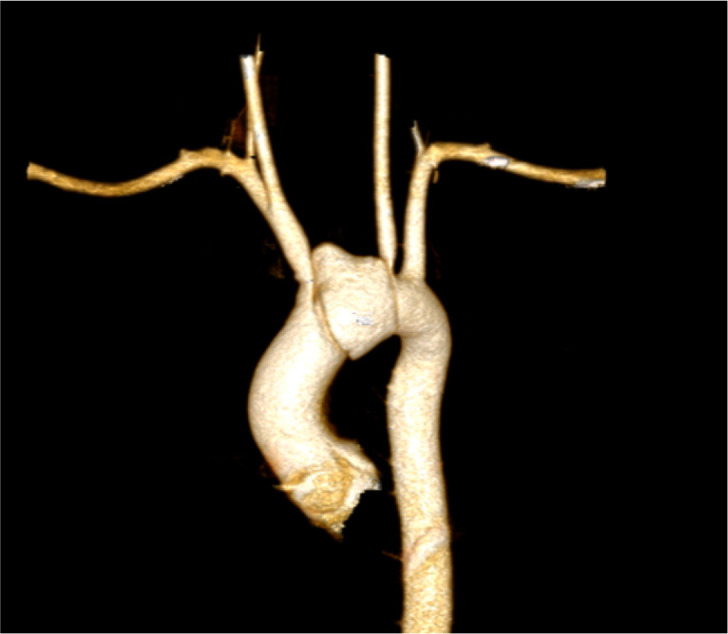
Preoperative CT scan (VRT reconstruction).
